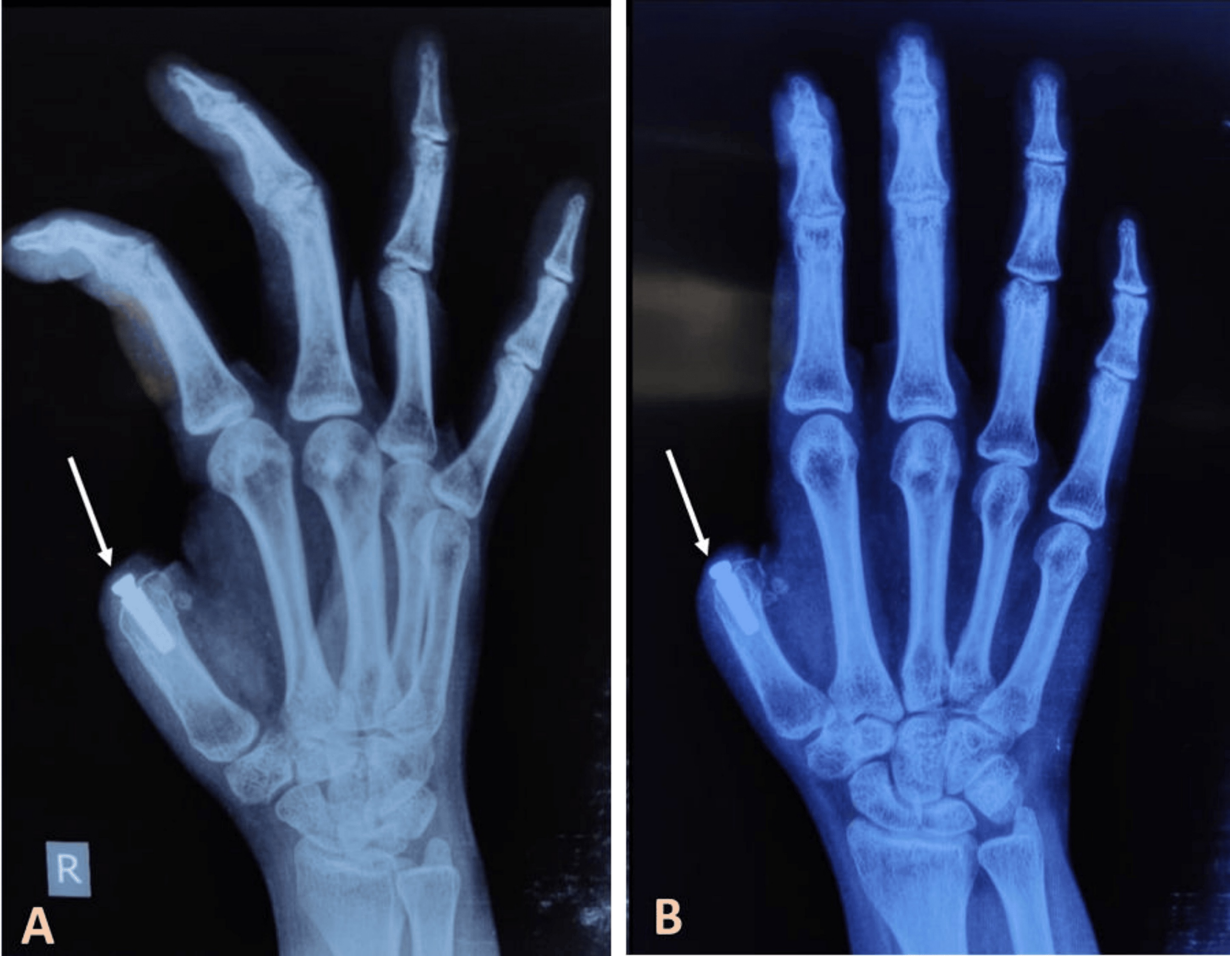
(A) Oblique- and (B) anteroposterior-view radiographs of the right thumb showing an osseointegrated implant (arrows)
